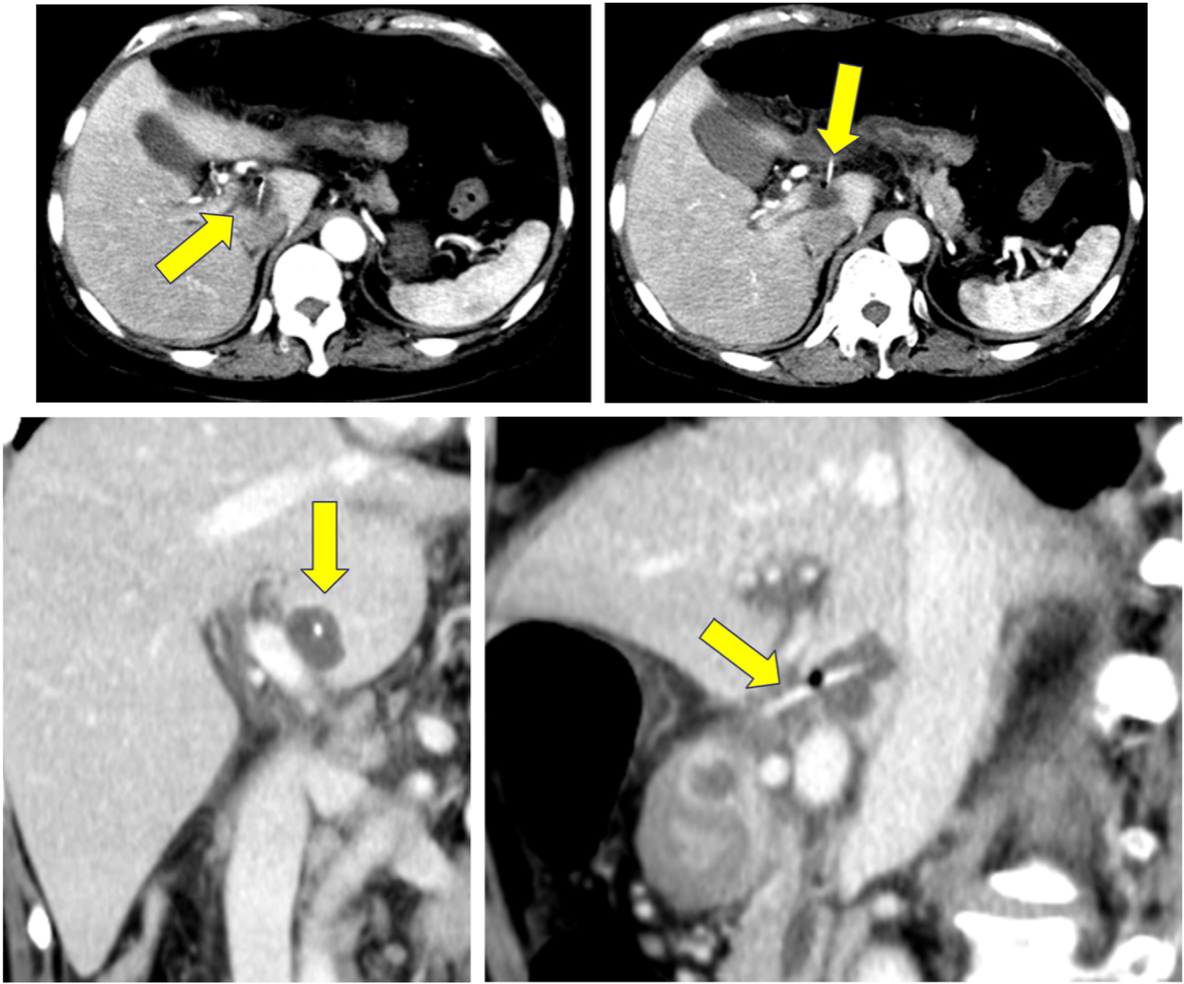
Enhanced computed tomography (CT) revealed a mass with ring enhancement (35 × 30 mm) and a needle-like foreign body. The distance between the tip of the foreign body and inferior vena cava was only 7 mm
